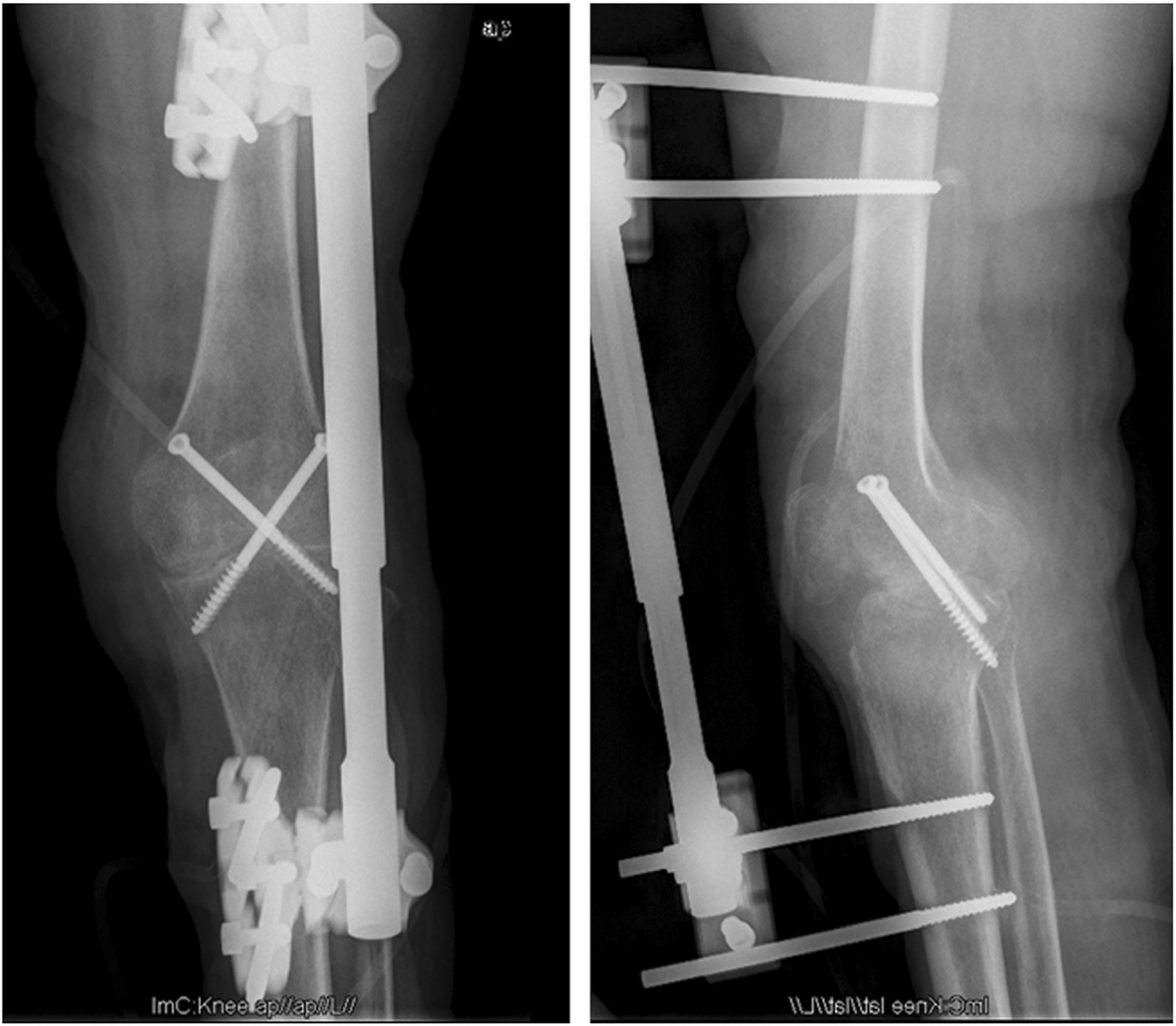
Good bony contact shown on radiographs (anteroposterior and lateral) after operation
